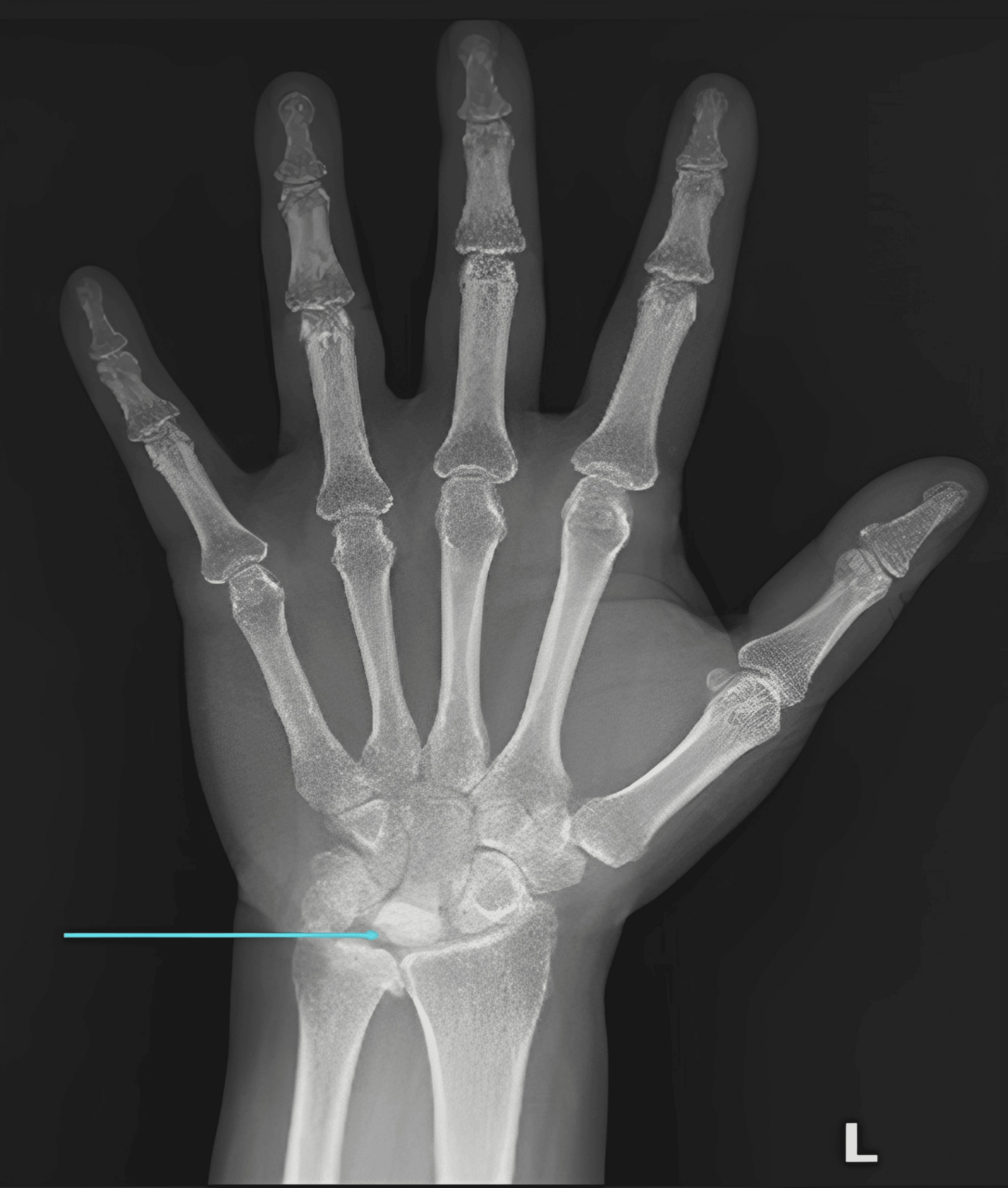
AP radiograph of the left hand showing a partially collapsed and sclerotic lunate (arrow), suggesting Kienbock’s disease
